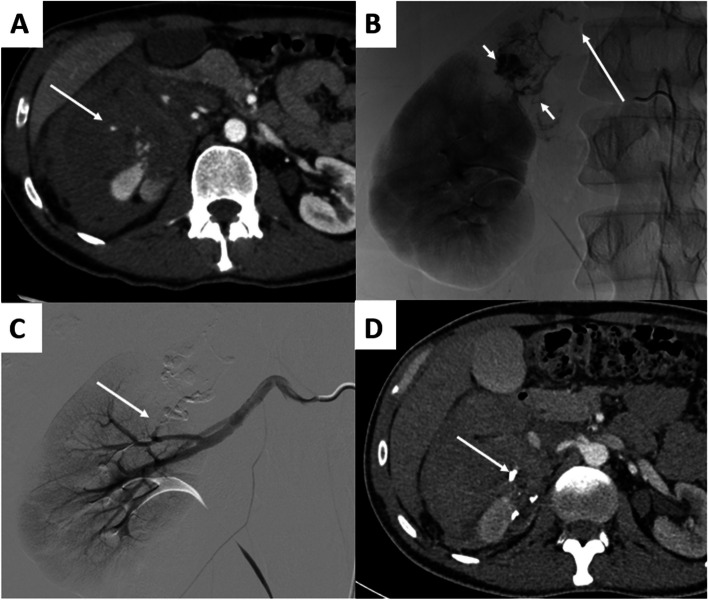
A 45-year-old patient presented with spontaneous right lumbar pain in the context of a known angiomyolipoma. A abdominal CT scan in axial section at arterial phase showed active bleeding with a large retroperitoneal hematoma (arrow). The tumor lesion was not distinctly visualized. B Angiography revealed tumor hypervascularization (short arrows) and active bleeding (long arrow). C After embolization with NBCA/lipiodol mixture (ratio 1/1), the angiography showed no active bleeding and no tumor opacification (arrow). D The CT scan performed 5 days later shows a reduction in the size of the retroperitoneal hematoma and lipiodol deposit (arrow)
